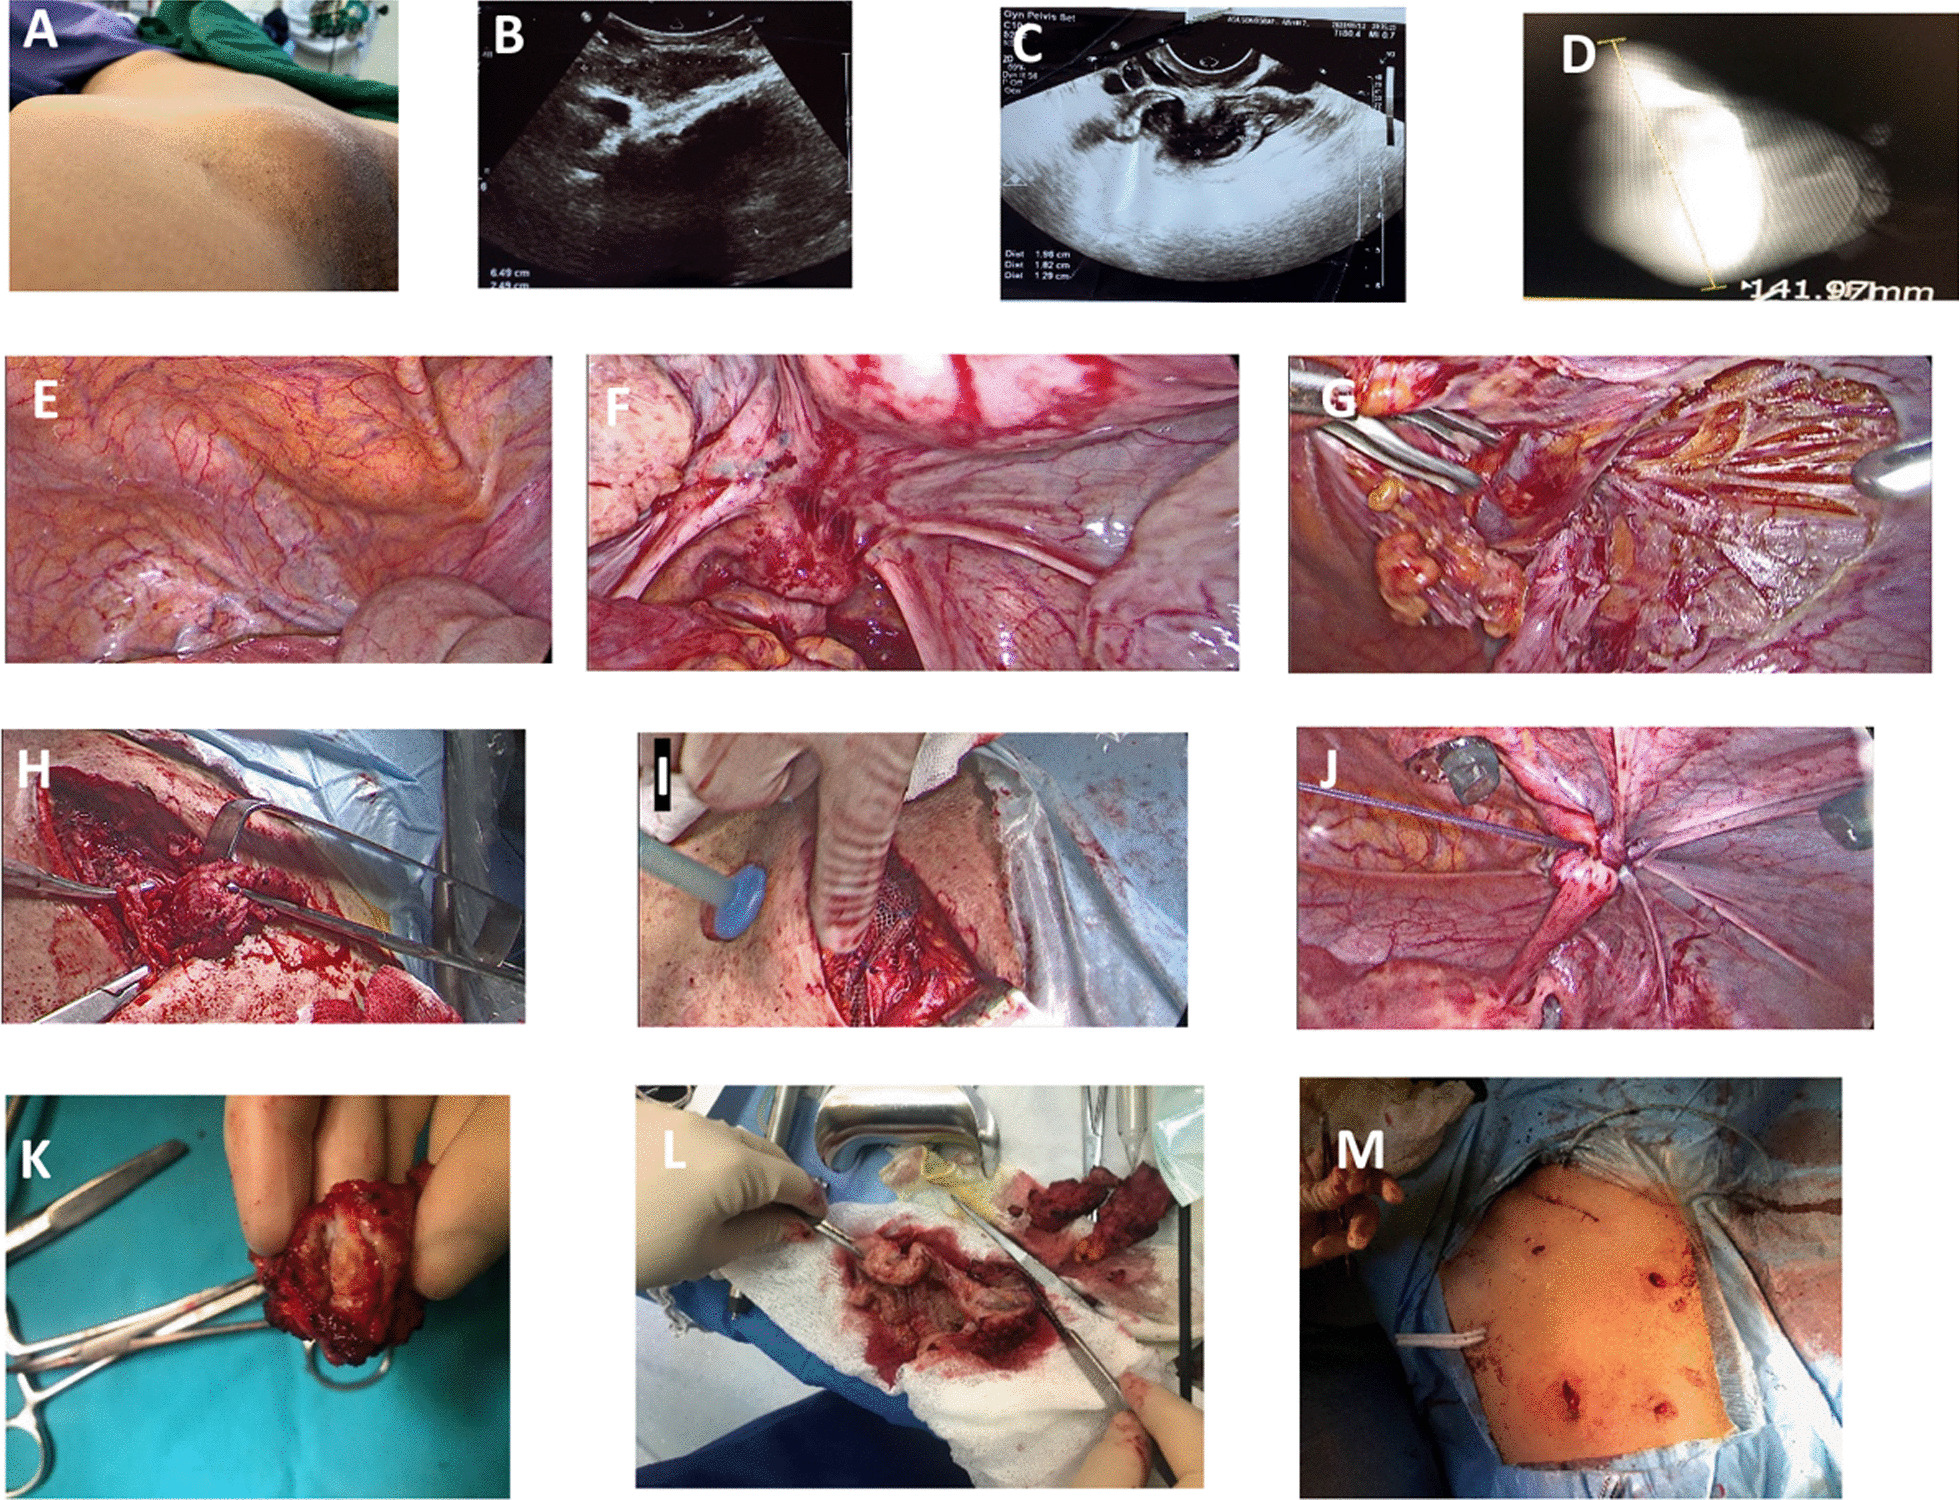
Pre-operative imaging assessment and intraoperative findings of case 5. A groin palpable mass; B inguinal endometriosis in ultrasound; C rectum endometriosis in transvaginal ultrasound; D inguinal endometriosis in magnetic resonance imaging; E inguinal bulging was seen during abdominal exploration; F uterosacral ligament endometriosis; G round ligament, external iliac artery, and vein after making incision; H pulling out inguinal mass via skin incision after resection with safe margin; I placing PROLENE Mesh on the external orifice of the inguinal canal; J peritoneal defect repairment; K inguinal endometriosis; L rectum endometriosis and inguinal mass; M Hemovac drain on inguinal site and corrugated drain on the anastomosis
